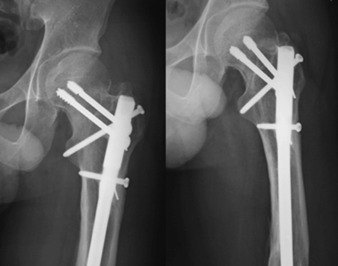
Plain radiographs of the patient shows radiological union at one month after the Miss-A-Nail technique screw fixation for the femoral neck fracture. The patient is able to return to full activity at two months after the surgery.
